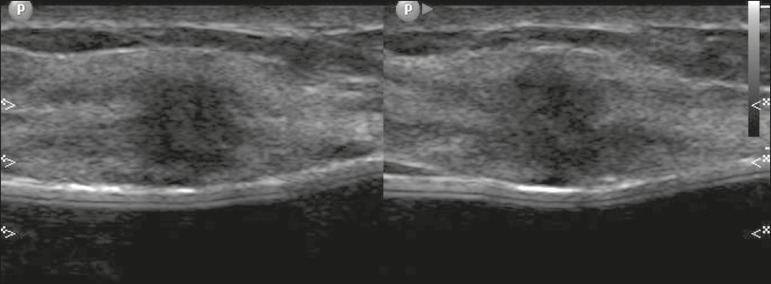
A 33-year-old patient at 27 weeks of pregnancy presented with a palpable mass in the left breast. Ultrasound showed a hypoechoic mass with irregular shape and non-circumscribed margins. A percutaneous biopsy confirmed the diagnosis of invasive ductal carcinoma.
